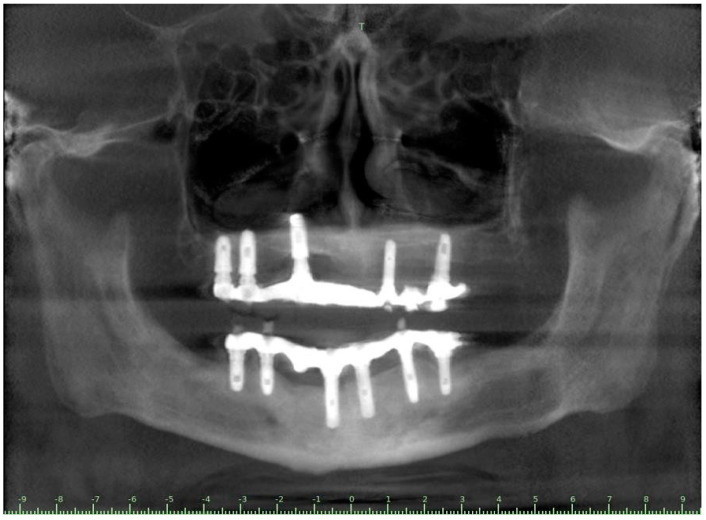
CBCT Panorex projection of the clinical case at the follow up.
